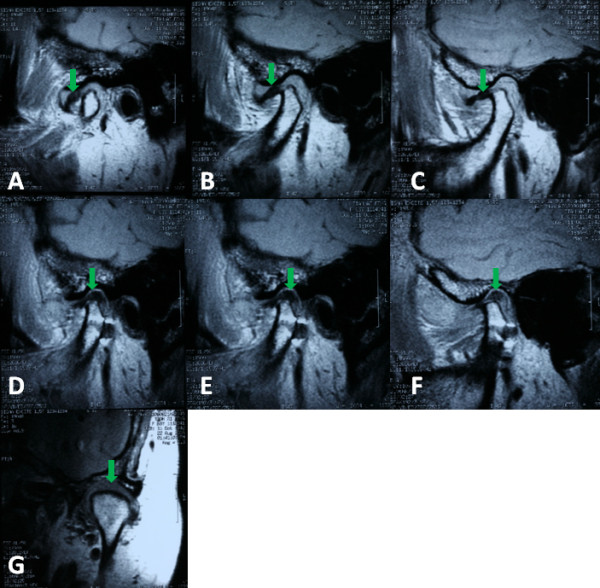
The disc position on MRI before and after operation. A, B, C showed the displaced disc anterior to the condyle (green arrow). D, E, F, G showed the displaced disc repositioned in normal position (green arrow)
